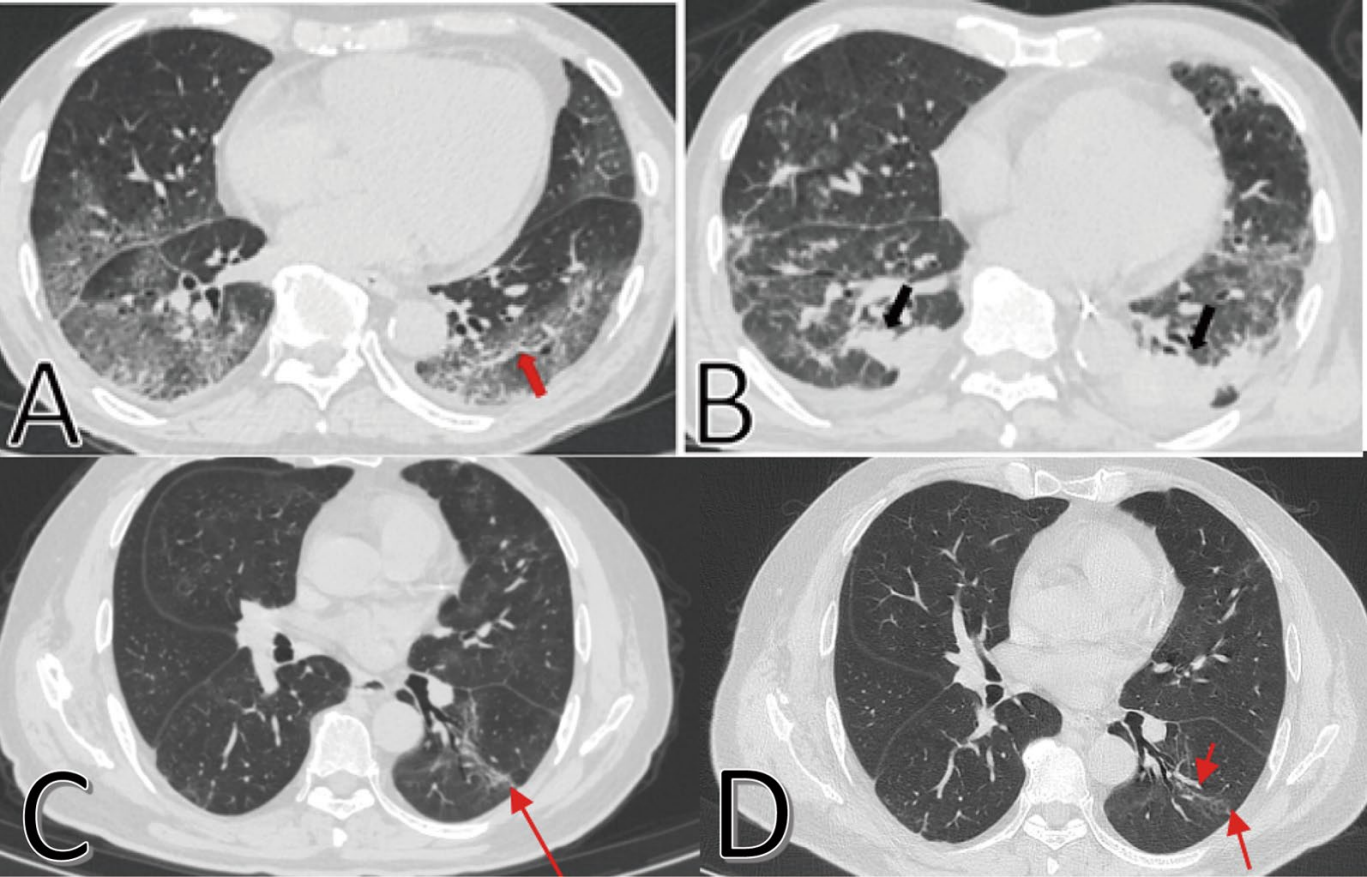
Typical CT imaging findings of a 67-year-old man with persistent pulmonary fibrosis. A Thin-section chest CT scan in our hospital on January 26, 2020 (9 days after symptoms onset). Chest CT imaging showed diffuse ground-glass opacities in both lungs, and a visible parenchymal band in the lower lobe of left lung (red arrow). B On March 23, 2020 (66 days after symptoms onset), diffuse ground-glass opacities were resolved partially in both lungs and new consolidation was observed. Irregular interfaces (black arrows) with a small amount of pleural effusion were observed in the lower lobes of both lungs. C On April 21, 2020 (95 days after symptoms onset), parenchymal bands and traction bronchiectasis were observed (red arrow). D On Jun 15, 2020 (150 days after symptoms onset), parenchymal bands and traction bronchiectasis were still observed in left lower lungs
